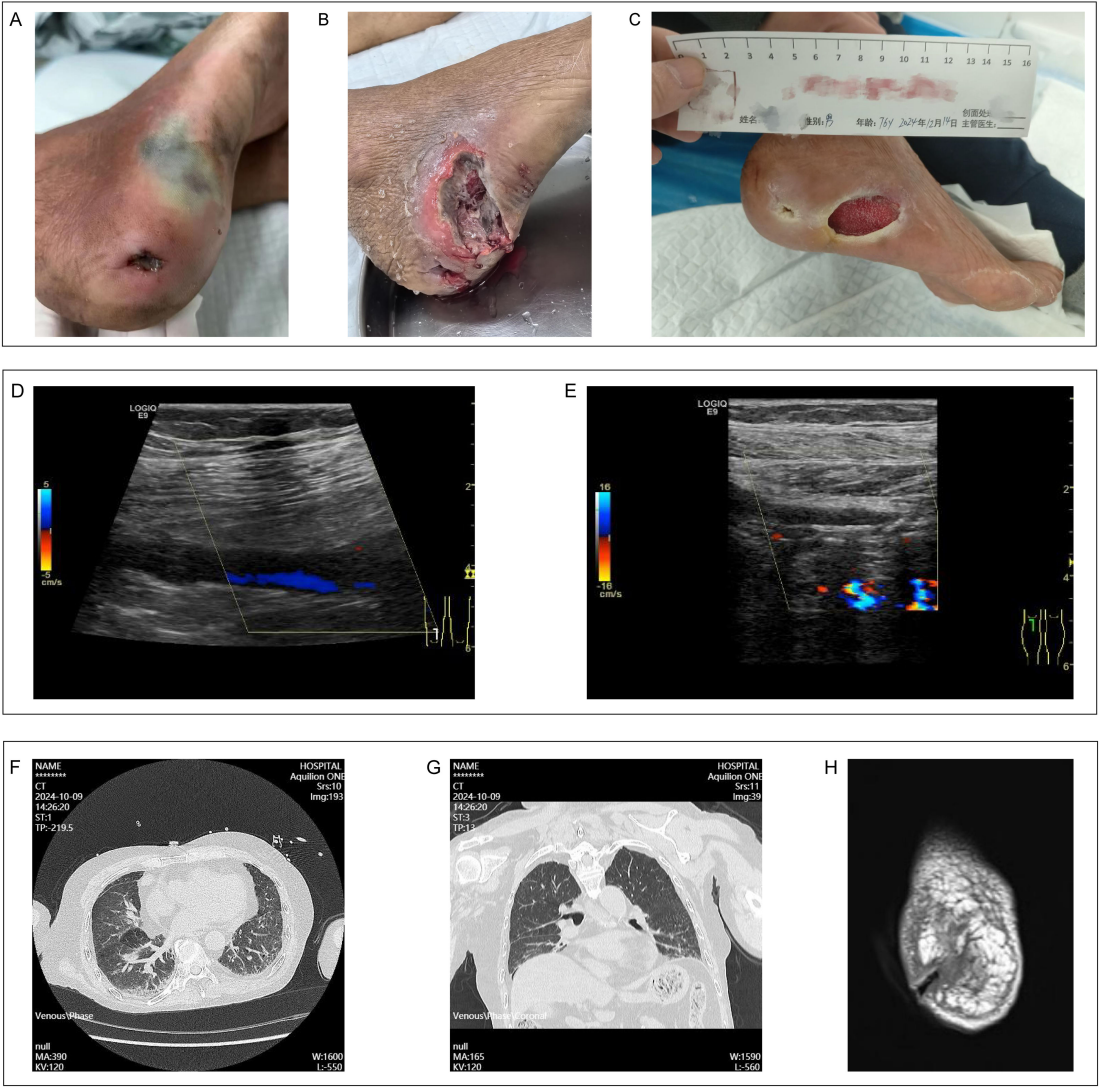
Clinical data for patient. (A) The condition of the wound on the patient's right foot admission. (B) The status of the right foot wound following incision and disinfection. (C) The healing process of the patient's right foot wound. (D) Color Doppler ultrasound imaging of thrombosis in the popliteal vein of the right lower limb. (E) Color Doppler ultrasound imaging indicating stenosis in the popliteal artery of the right lower limb. (F, G) Chest CT findings obtained subsequent to the patient's admission. (H) MRI findings pertaining to the wound on the right foot upon the patient's admission.
